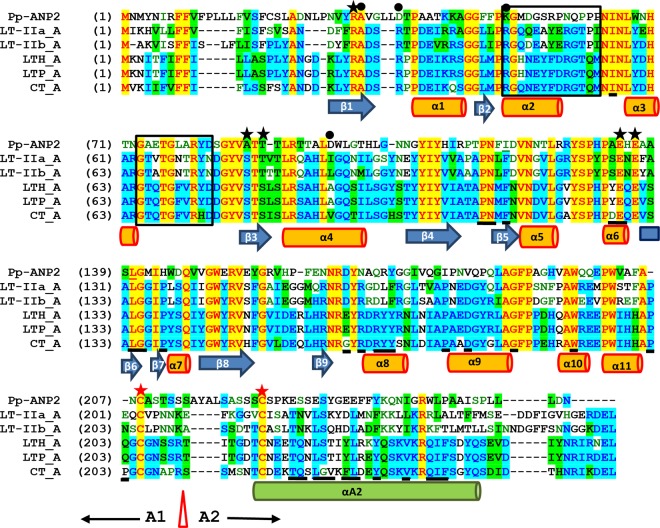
Protein sequence alignment of Pp-ANP2a and subunit A of CT/LT toxins. Blue arrow bars indicate β-sheet regions and orange cylinders α helical regions. Two boxes indicate the two activation loops. Red stars show the conserved cysteines and the red triangle shows cleavage sites in CT and LT toxins that cleave the protein into domains A1 and A2. Green cylinder shows the α helix in domain A2. Black stars highlight the highly conserved catalytic motifs (R/H-STS-ExE) across the ADPRT superfamily while black dots indicate additional NAD interacting residues. Underlined letters in the CTA sequence indicate A1 and A2 interface residues in the nonreduced/disulfide-bonded CTA form.
